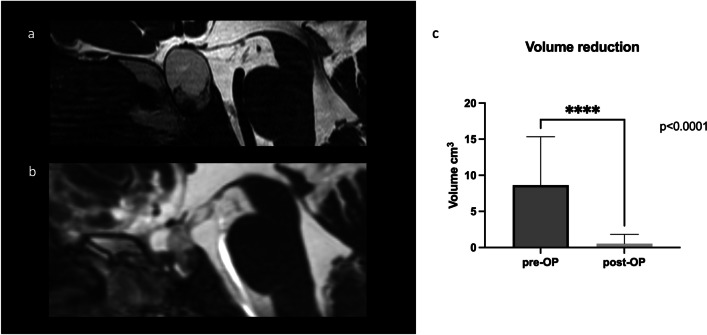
Exemplary sagittal T2 MRI before (a) and after (b) transnasal transsphenoidal decompression of RCC (c) shows a significant reduction in cyst volume by surgery in the entire patient cohort
